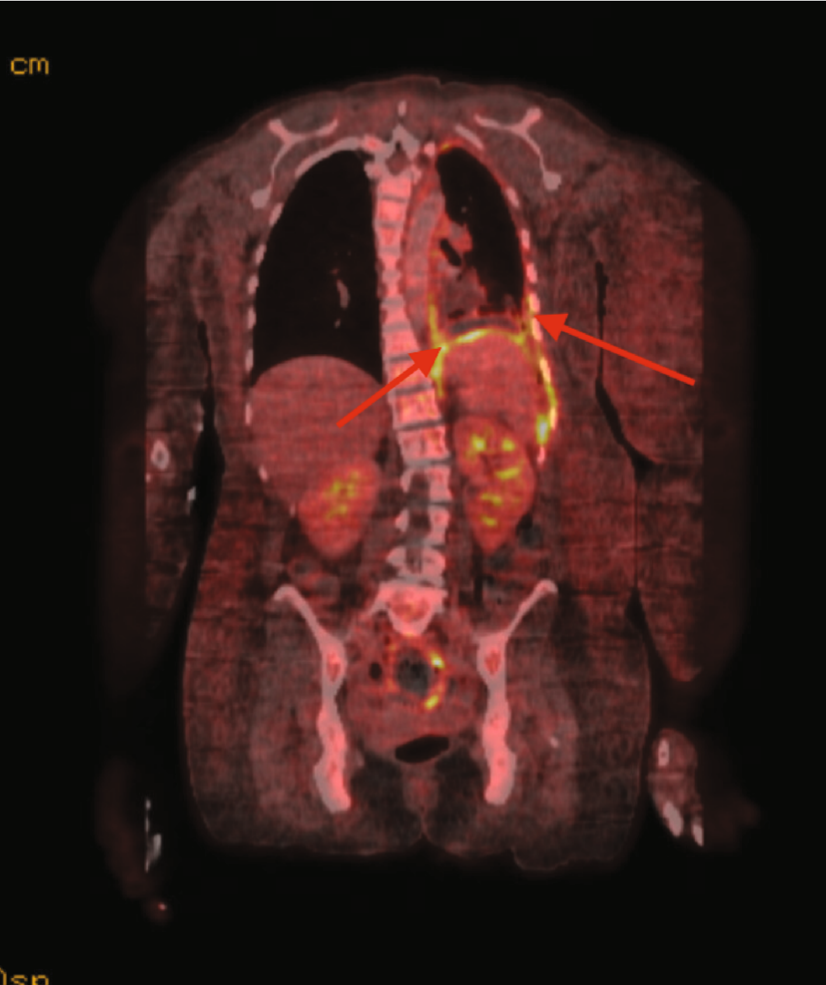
PET-CT (coronal). Diffuse pleural thickening with high activity encapsulating the entire left lung.
